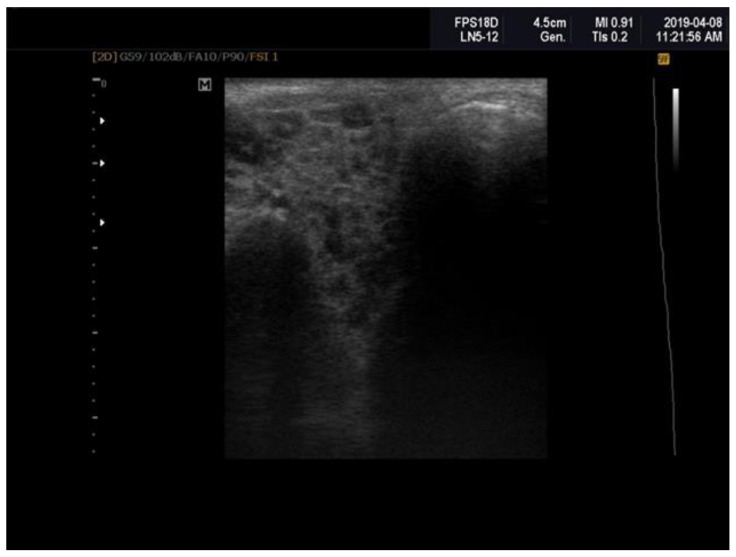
Sjögren’s syndrome; parotid ultrasonography; Hocevar score = 12.
